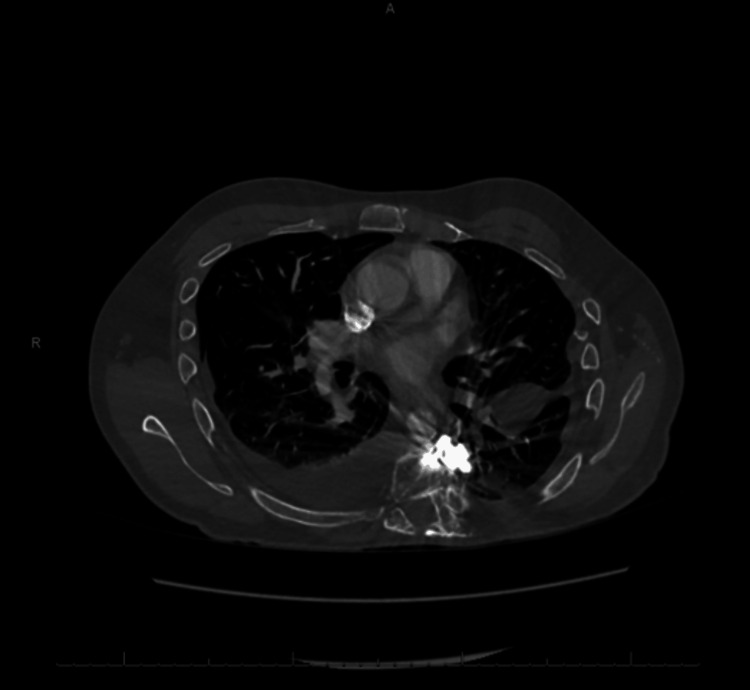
CTA of the chest revealing the fusion orthopedic hardware component in the anterior prevertebral space abutting the pleura (axial view) CTA: computed tomography angiogram
